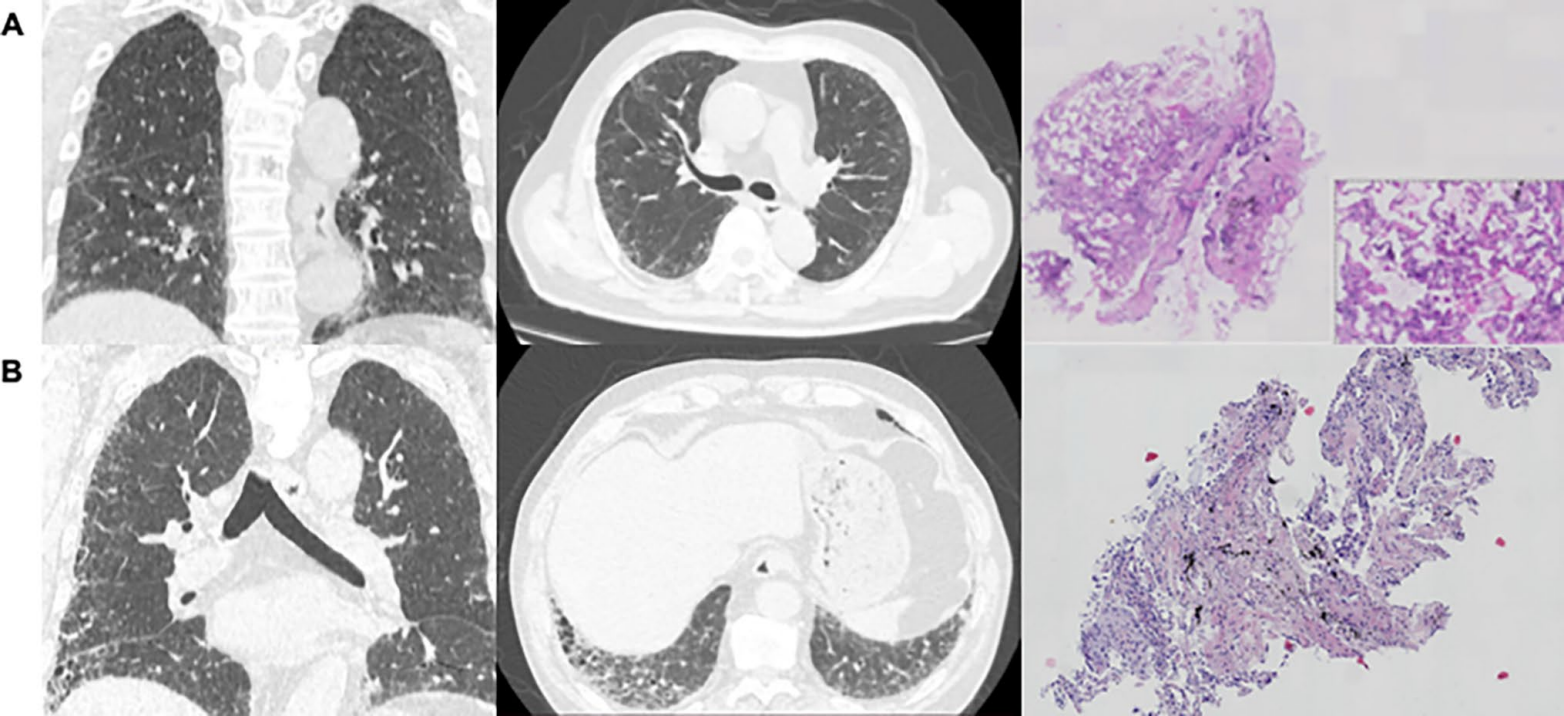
A A patient with a definite diagnosis of nonspecific interstitial pneumonia. CT showed non-fibrotic presentation of large bilateral pulmonary ground glass opacity with bilateral lower lung basal reticular pattern. Medium magnification of the lung showed lung tissue focal alveolar septum widening with lymphocyte and plasma cell infiltration, focal multinucleated giant cells, and alveolar epithelial hyperplasia, and no granulomas were seen. B A patient with a definite diagnosis of idiopathic pulmonary fibrosis. CT demonstrated fibrotic presentation of subpleural reticular pattern and ground glass opacity with honeycombing changes at the base of both lungs. Low magnification of the lung showed some of the alveolar septa widened with a little lymphocyte and plasma cell infiltration and alveolar epithelial hyperplasia; macrophages were seen in the alveolar lumen
